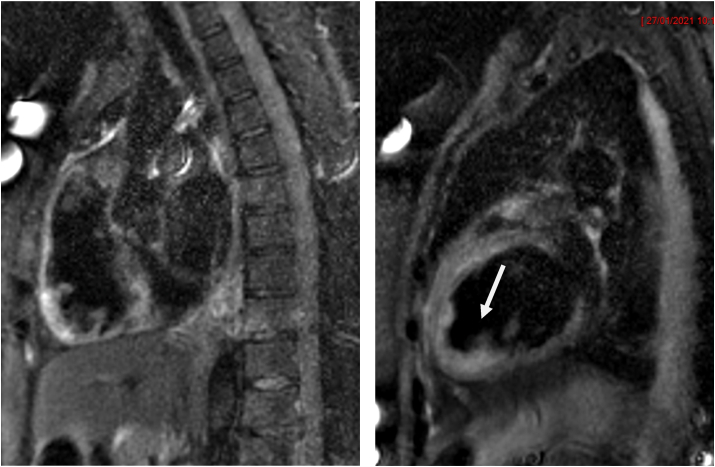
T1-weighted sagittal sequence (10 minutes after injection of contrast product): focal thickening (arrow), with an exaggerated enhancement compared to the rest of the myocardium.
